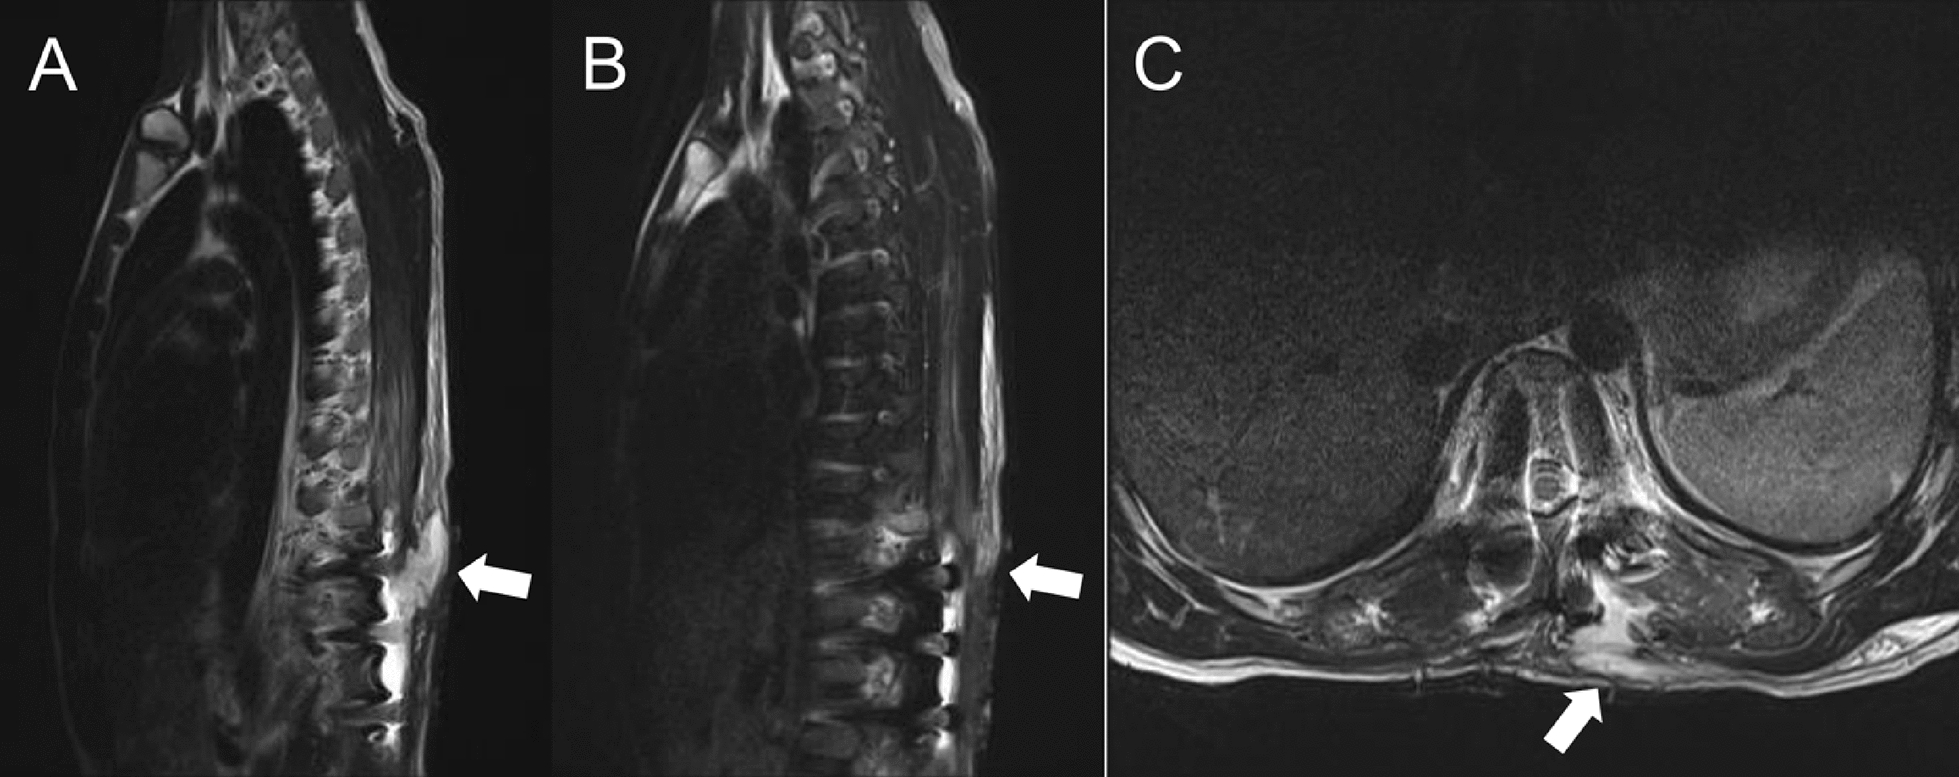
Magnetic resonance imaging visualization of postoperative fluid accumulation in the paraspinal soft tissues. A, B Sagittal fat-saturated T2-weighted magnetic resonance imaging sequences of the thoracolumbar spine demonstrate an asymmetric hyperintense fluid collection on the left side (A, arrow) involving subcutaneous and intramuscular compartments, compared with the appearance of the contralateral (right) side (B). C An axial T2-weighted magnetic resonance image through the T12 level localizes the fluid collection (arrow) to the left paravertebral musculature. These imaging characteristics are consistent with a postoperative seroma (sterile fluid accumulation) or reactive edema in the soft tissues
